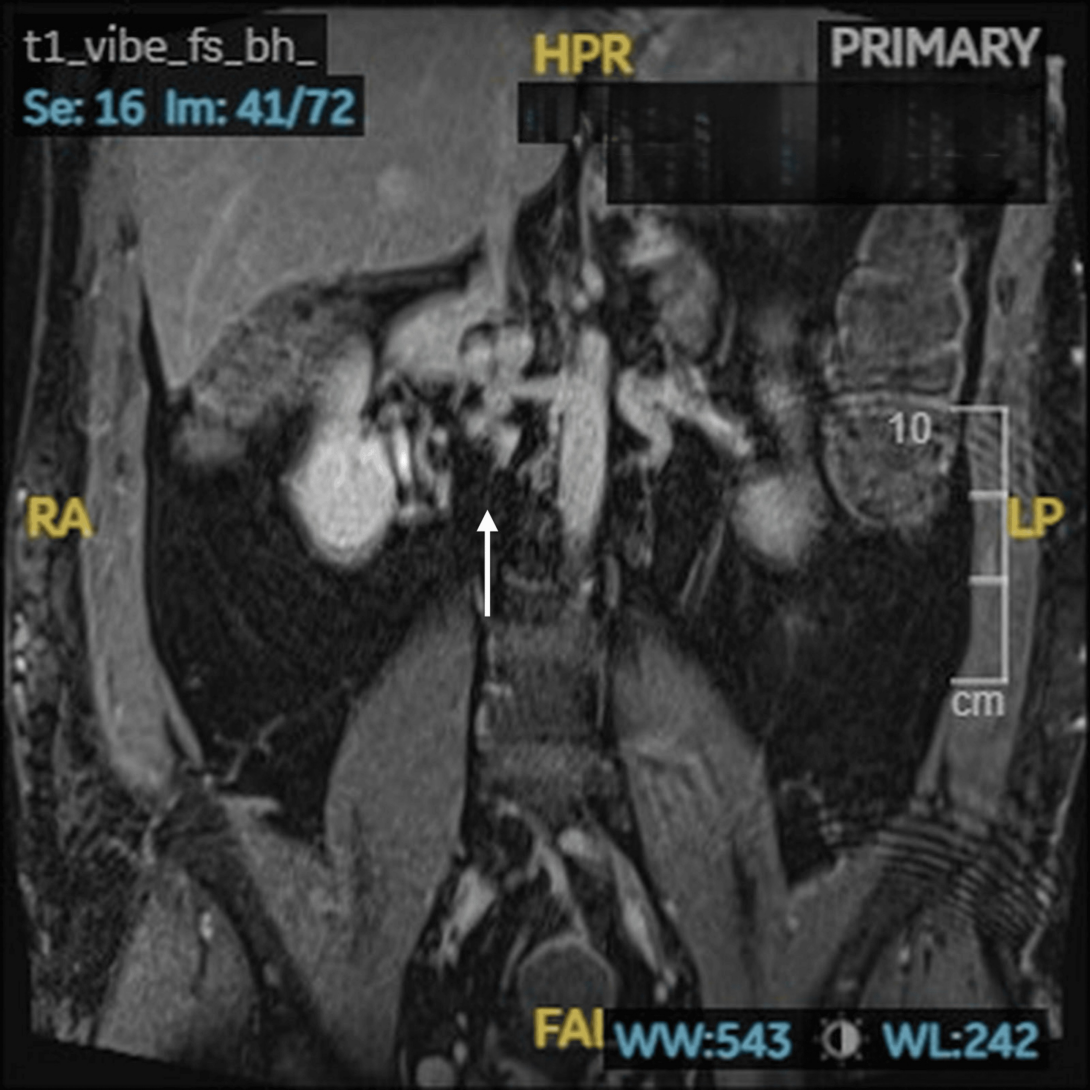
Nonvisualization of the infrarenal inferior vena cava. Coronal view of contrast-enhanced MRI of the inferior vena cava demonstrating nonvisualization of the infrarenal inferior vena cava.
